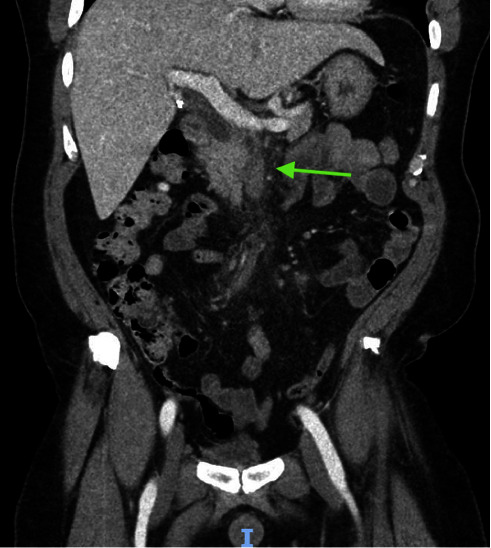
Coronal section obtained from CT of the abdomen and pelvis with intravenous contrast demonstrates superior mesenteric vein thrombosis with surrounding inflammation consistent with thrombophlebitis (green arrow).
